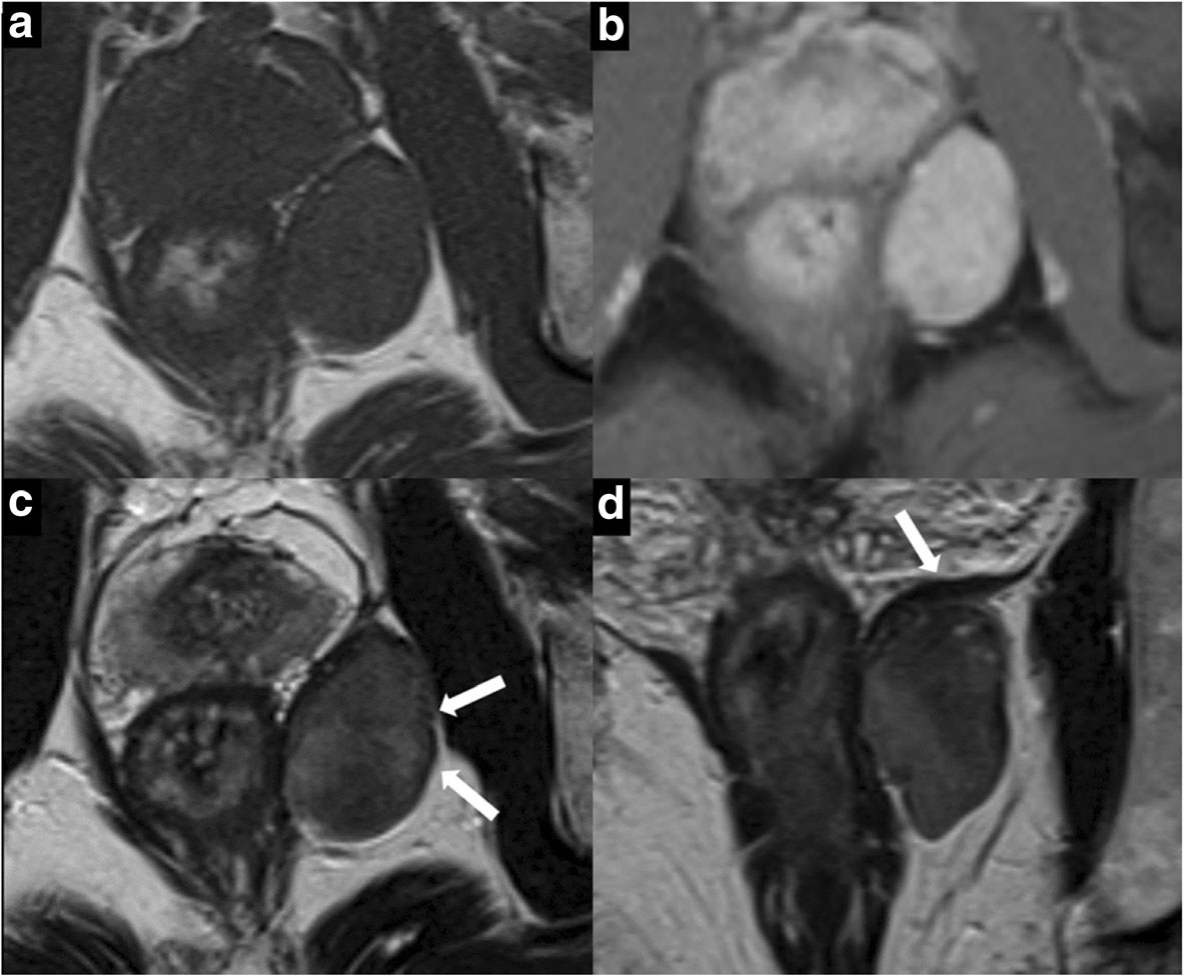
MRI findings. a Axial T1-weighted image shows homogenous intermediate signal intensity. b Gadolinium contrast-enhanced fat-suppressed T1-weighted image (4 min after contrast material injection) shows homogenous enhancement. c Axial T2-weighted image shows heterogeneous intermediate and low signal mixed intensity. The mass contains areas of low signal bands and heterogeneous high signal intensity (white arrows). d Coronal T2-weighted image: the tumor is present under the levator ani muscle (white arrow)
